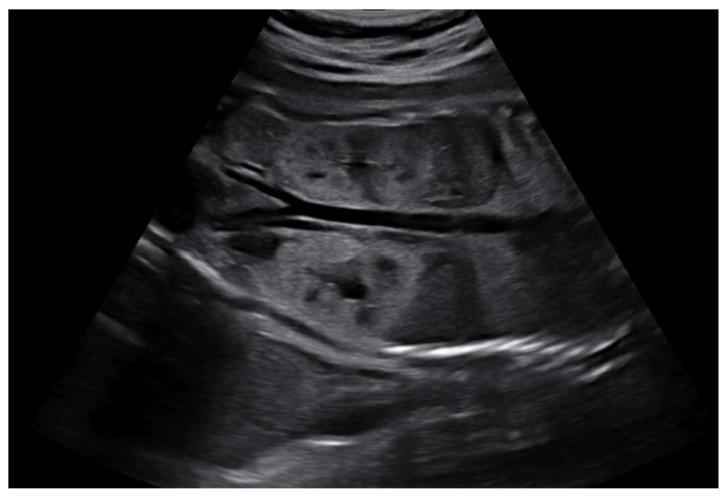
Sonographic findings of fetal ADPKD (Case 14). Ultrasound image of the first fetus of a monochorionic diamniotic twin pregnancy at 24 weeks’ gestation, demonstrating typical features of ADPKD. The kidney is enlarged above the 97th percentile, with a hyperechoic cortex and increased corticomedullary differentiation. Second fetus of the twin pregnancy showed same findings and diagnosis.
